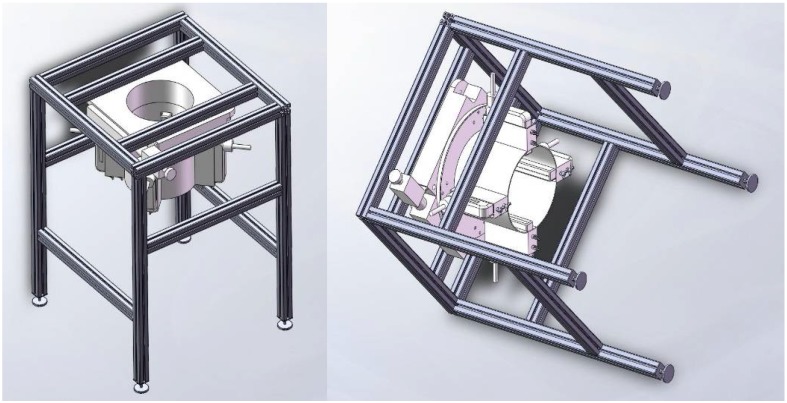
Experimental setup for the 3D ultrasound imaging ring system.
